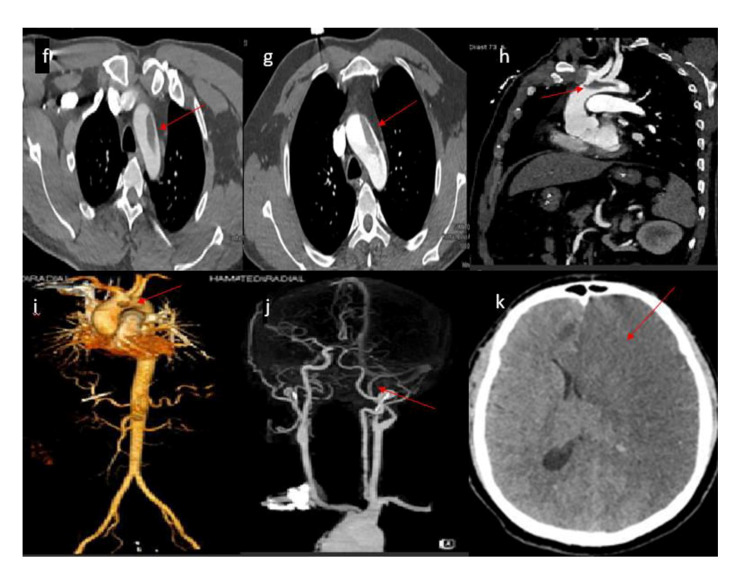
CT angiography showed a large 5 x 1 cm floating thrombus in the arch of the aorta attached to the left anterolateral wall (f-i). CT angiography showed left ICA occlusion petrous and inter-cavernous portion and significant attenuation of the M1 and M2 portion of left MCA (j). CT head showed large left MCA acute ischemic stroke and a small right frontal parafalcine region and 7 mm midline shift to the right side with mass effect (k). MCA: Middle cerebral artery; ICA: Internal carotid artery.
